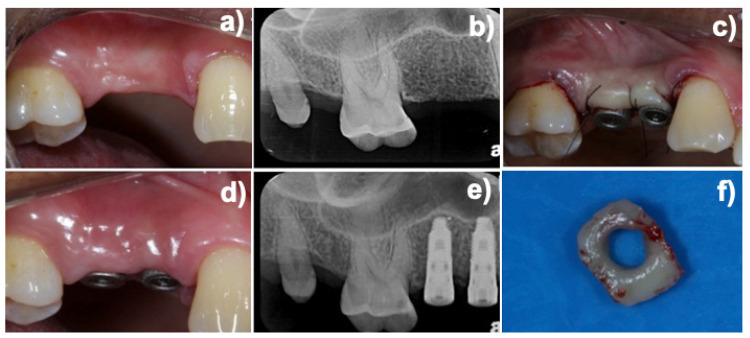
(a) View of the edentulous area before dental implant placement and (b) the radiographic preoperatory view. (c) Experimental healing connected to the implant after suture and (d) after 30 days of healing. (e) Radiographic view of the connected healing abutments and (f) soft tissue biopsy.
